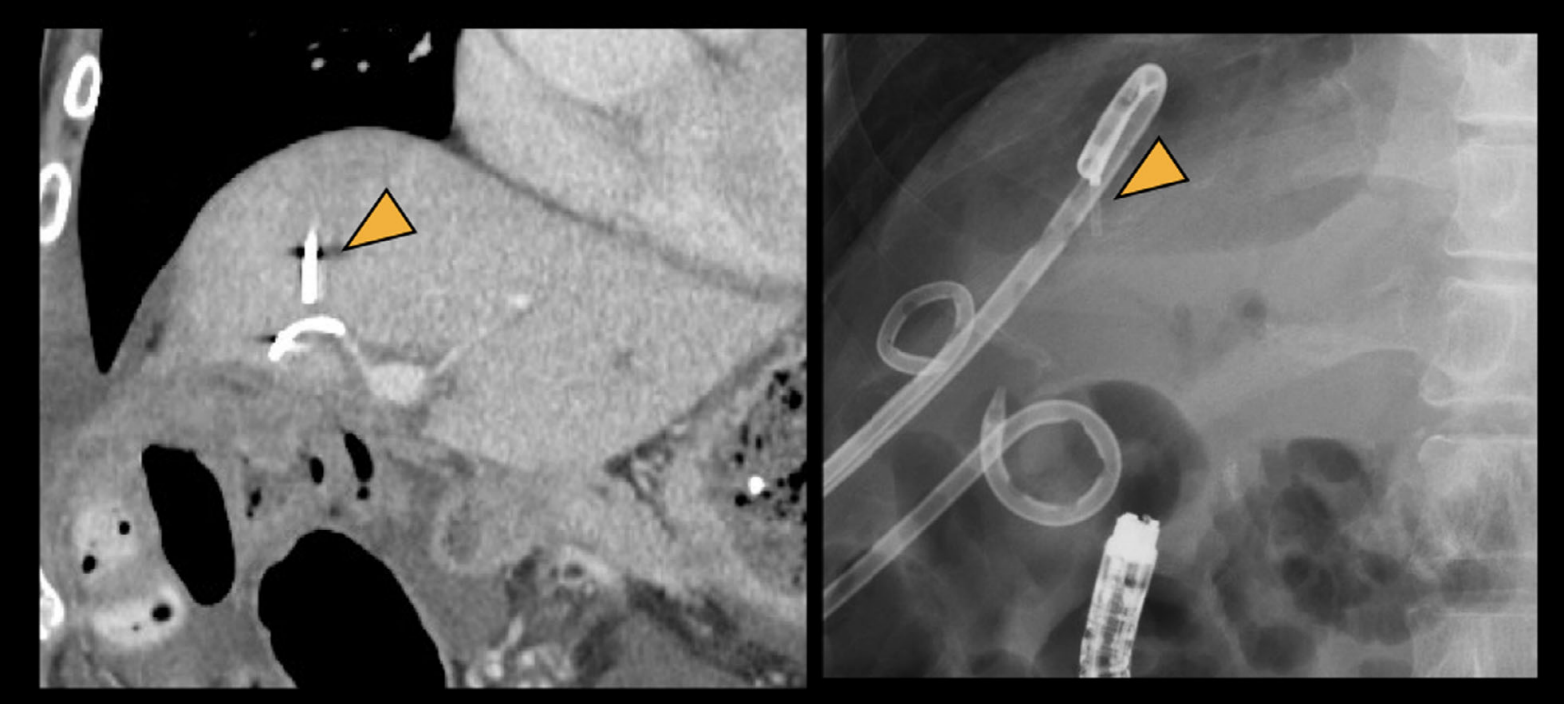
Computed tomography scan and radiograph showing a sheared nasobiliary drainage tube in an intrahepatic bile duct (arrowhead).
